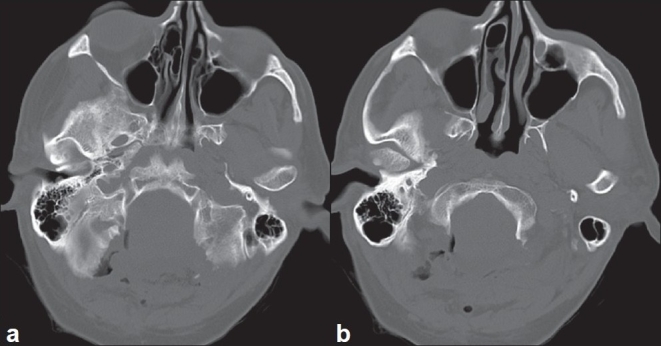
Postoperative axial computed tomography scans at slightly different cuts (a, b) showing the extent of bony decompression achieved. [Used with permission from Barrown Neurological Institute]
